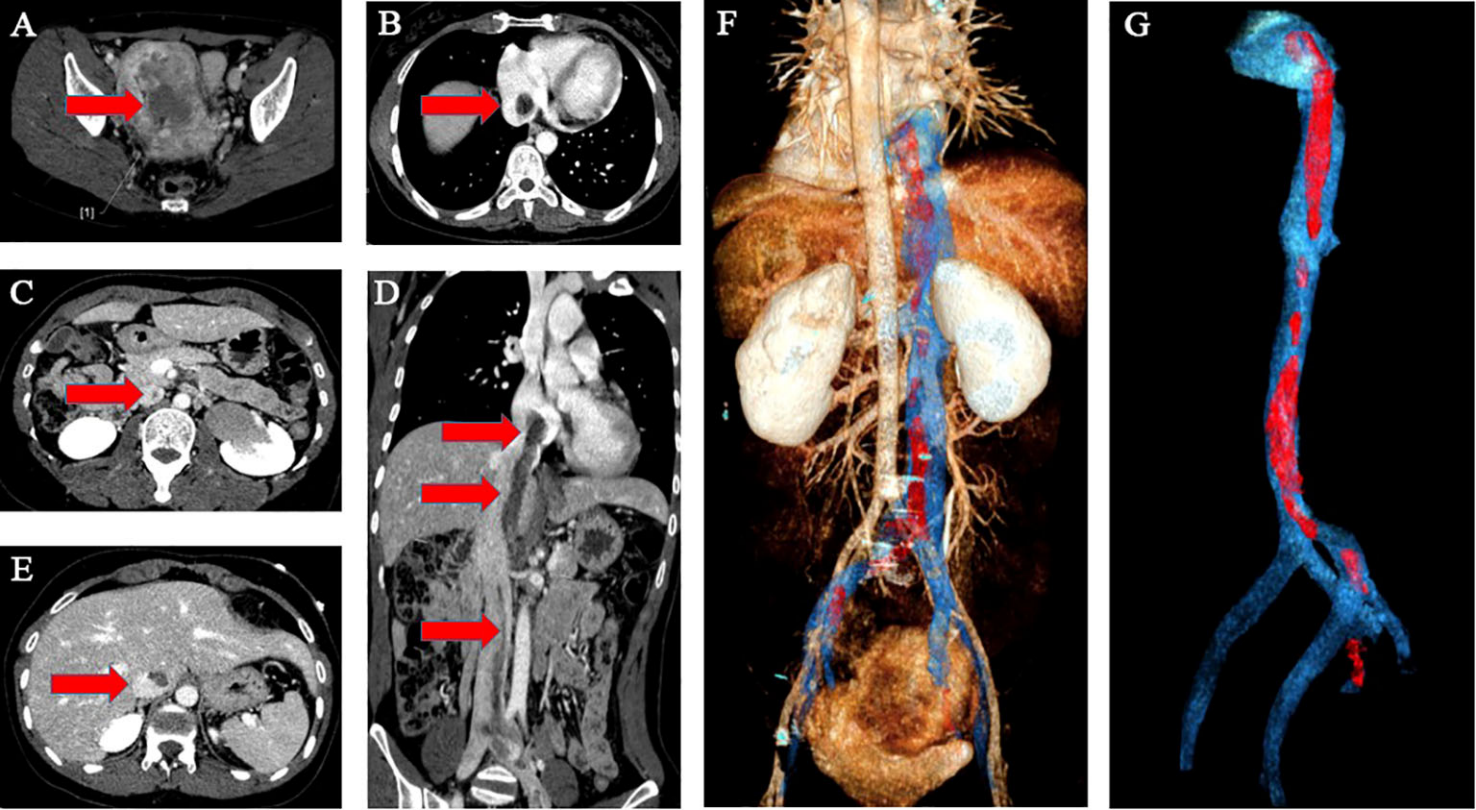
Imaging of the tumor. The superior and inferior vena cava CTA showed an enlarged uterine as well as low-density image in the venous system and the right atrium (A–E). The red arrow indicates the mass. The three-dimensional computed tomography reconstruction of the whole path of the mass originating from the uterine and invaded into the venous system and eventually to the right atrium (F, G). (F) Back view (G). Endovascular imaging of the tumor. Red represents the mass; blue represents the blood vessel.
